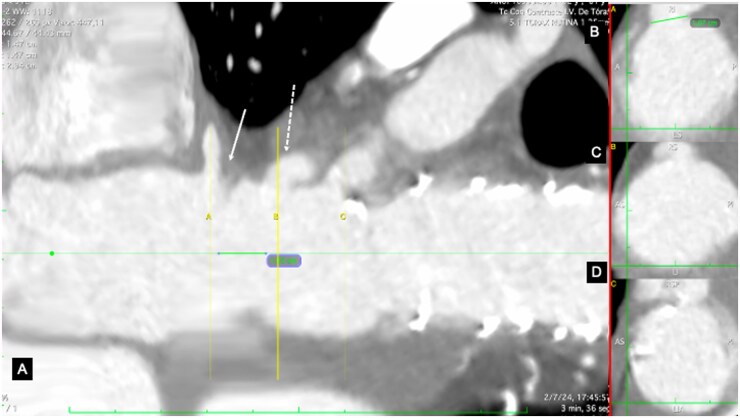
Imaging of Patient no. 2 with Prior Frozen Elephant Trunk. (A) CT Reconstruction Showing the Pseudoaneurysm at the Proximal Anastomosis and the Distance to the Translocated LCCA Bypass (Solid Arrow: entry Tear; Dashed Arrow: LCCA Bypass). (B–C) Alignment of the Entry Tear and LCCA Bypass. (D) Origin of the Translocated Brachiocephalic Trunk Bypass.
